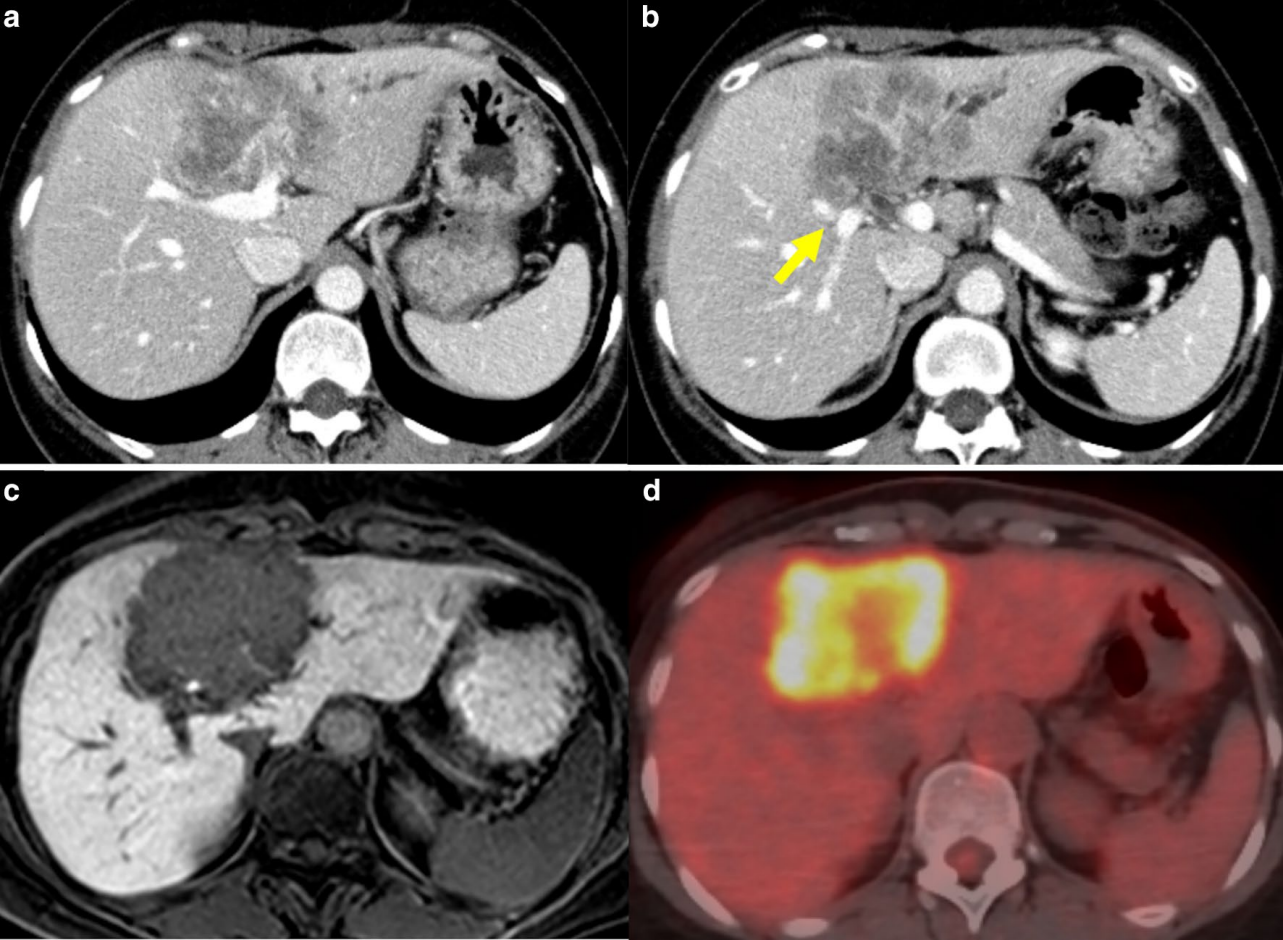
Images at admission of local hospital. a Contrast-enhanced abdominal computed tomography (CT) showed the tumor was mosaic enhancement, measured 65 × 61 mm in the hepatic segments 4 and 5 and 8. b Contrast-enhanced abdominal CT showed the tumor was attached to the biliary confluence, bifurcation of anterior–posterior Glissonian (yellow arrow) and right portal vein trunk. It was suspected to invasion to the left portal vein trunk, left intrahepatic bile duct and middle hepatic vein. c Magnetic resonance imaging (MRI) showed the tumor was hypointense on T1-weighted images. The margin of the tumor was unclear. d
